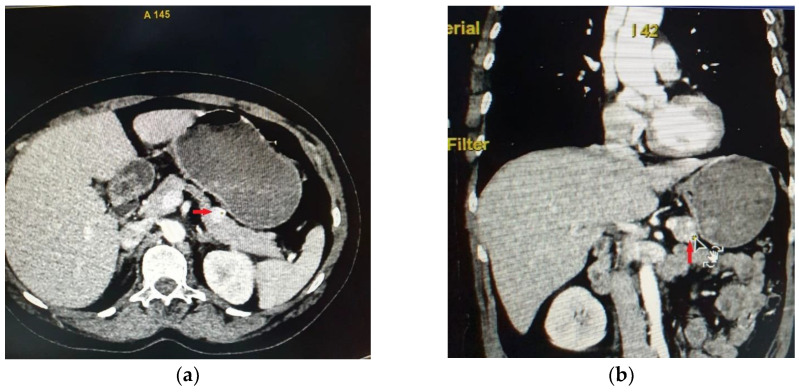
Abdominal CT-axial section (a) and coronal section (b), indicating that in the anterior part of the pancreatic corporeo-caudal region, there is an isodense tumor measuring 15.3/15 mm with intense iodophilia.
